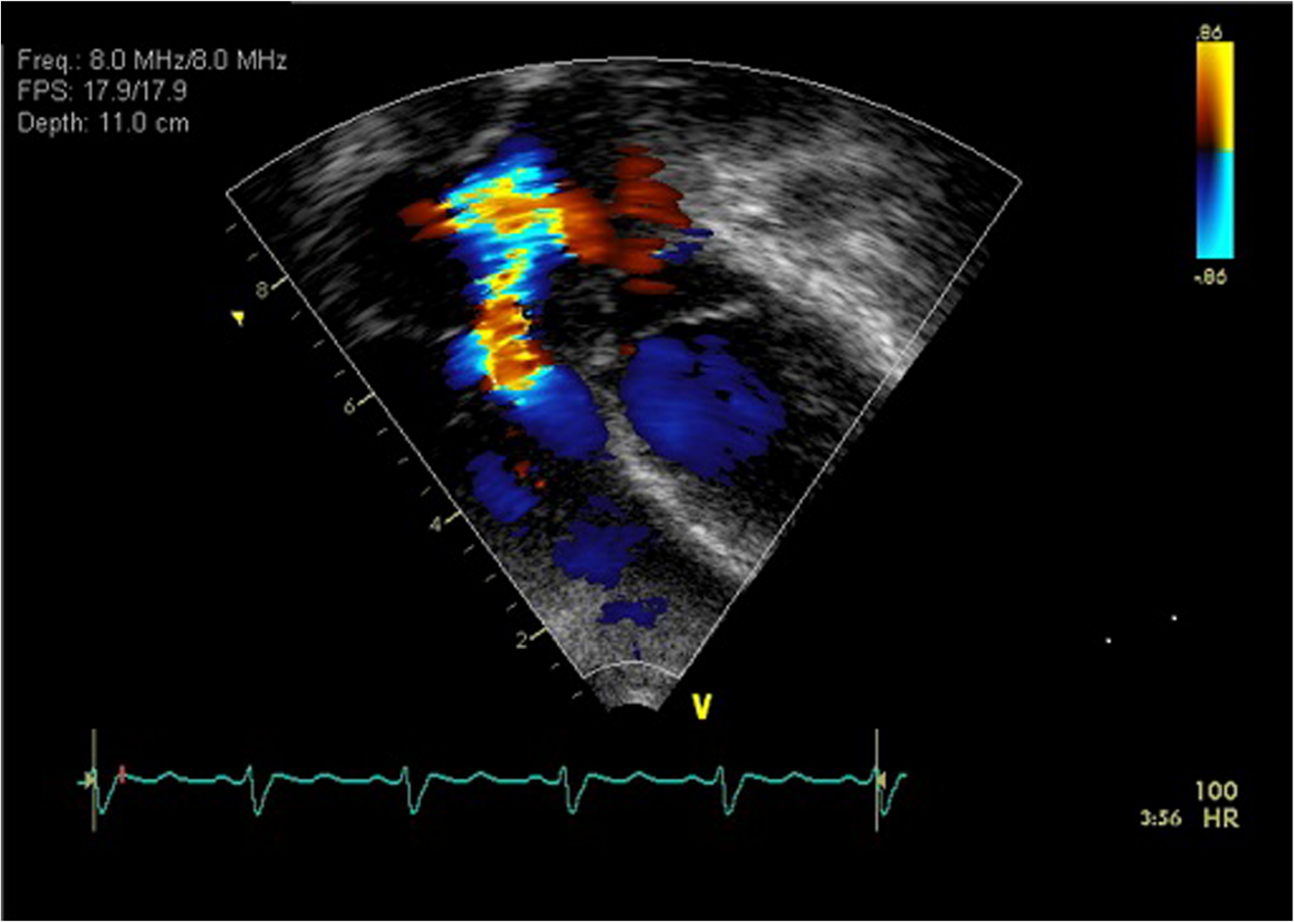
An echocardiographic image demonstrating an incomplete tricuspid atresia with moderate regurgitation in the patient
